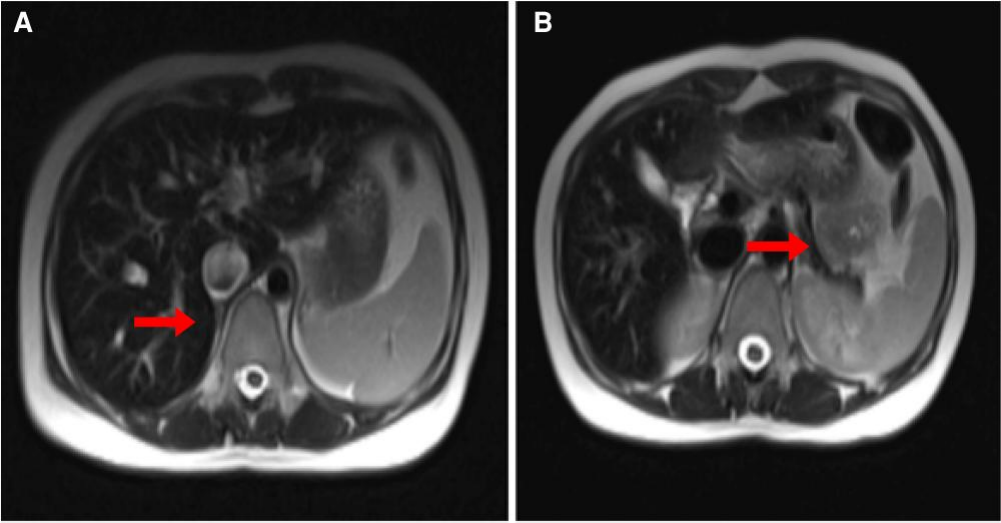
(A) Abdominal MRI showing hypointensity of the liver, suggesting iron deposition. (B) Abdominal MRI showing pancreatic hypointensity, consistent with pancreatic iron overload.
